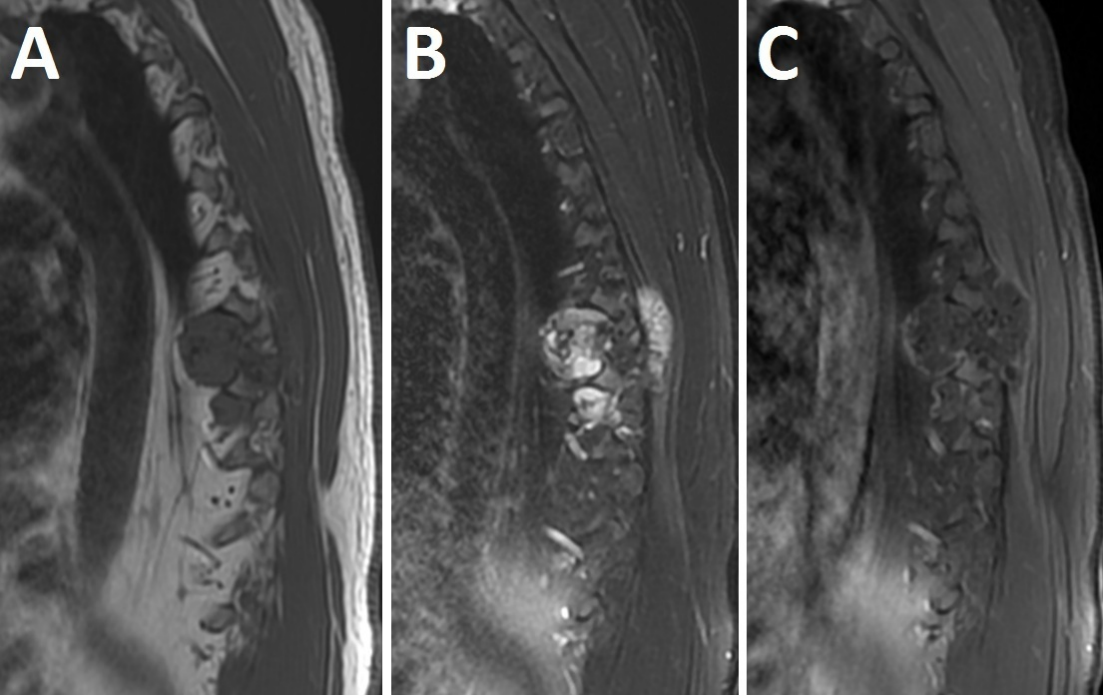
Preoperative MRI Preoperative T1-weighted (A), T2-weighted (B) and T1-weighted gadolinium (C) MRI showing a polylobulated, partially calcified, T2 hyperintense and minimally peripherally enhancing mass centered on the left costovertebral joint at the level of T7 with extensions within the left neuroforamina at T7-8 and T8-9.
